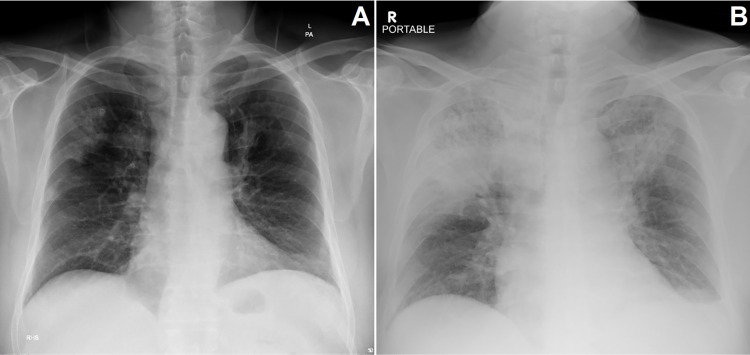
Chest x-ray: (A) posteroanterior view on the first day of admission; (B) anteroposterior view portable on the sixth day of admission The chest x-ray on presentation (A) shows limited and ill-defined patchy peripheral consolidations seen in the right upper and middle zones. There are increased bronchovascular markings bilaterally. No pleural effusion is seen. Mild elevation of the cupola is noted. The follow-up chest x-ray performed six days later in the ICU (B) demonstrates bilateral extensive upper and middle zone consolidations predominantly in the right lung with reduced lucency of the remaining lung fields. Blunting of the left costophrenic angle is observed.
